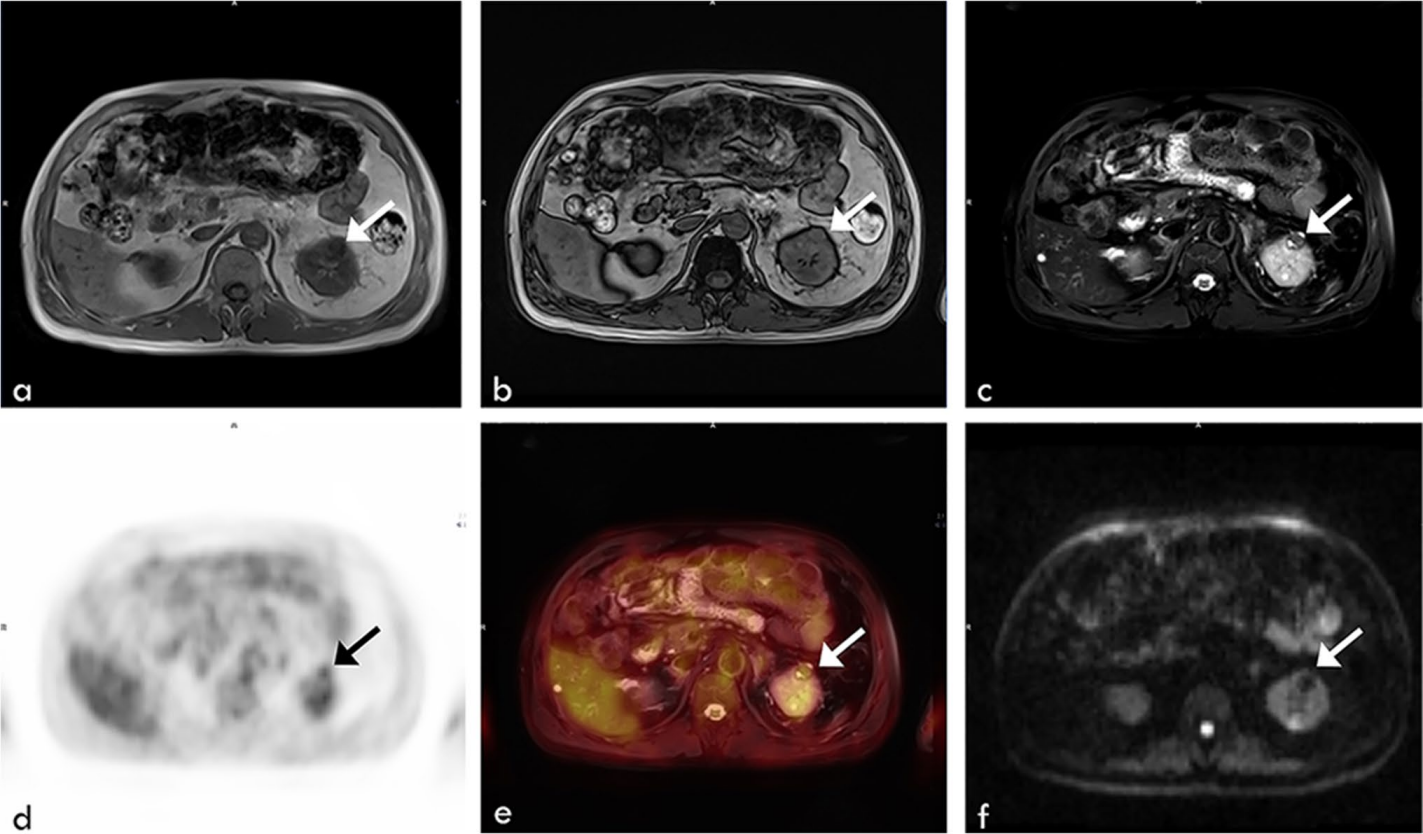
Axial PET/MRI images of a 65-year-old man with renal clear cell carcinoma. A lesion (arrow) was seen in the left kidney as an area of low signal intensity on the T1-weighted image (a, in phase; b, out of phase) and diffusion-weighted image (f), with cystic necrosis on the T2-weighted image (c). Mild FDG uptake was observed on the PET image (d) and on the fused T2-weighted and PET image (e)
